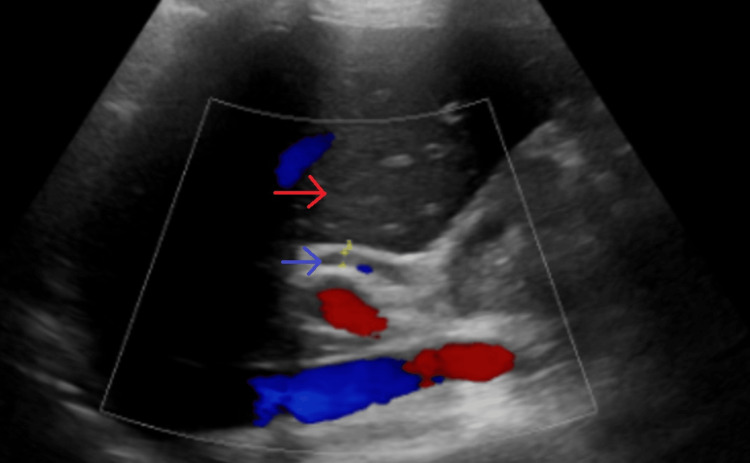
Ultrasound of the liver with normal texture (red arrow) and non-dilated common bile duct, 0.32 cm (blue arrow)
